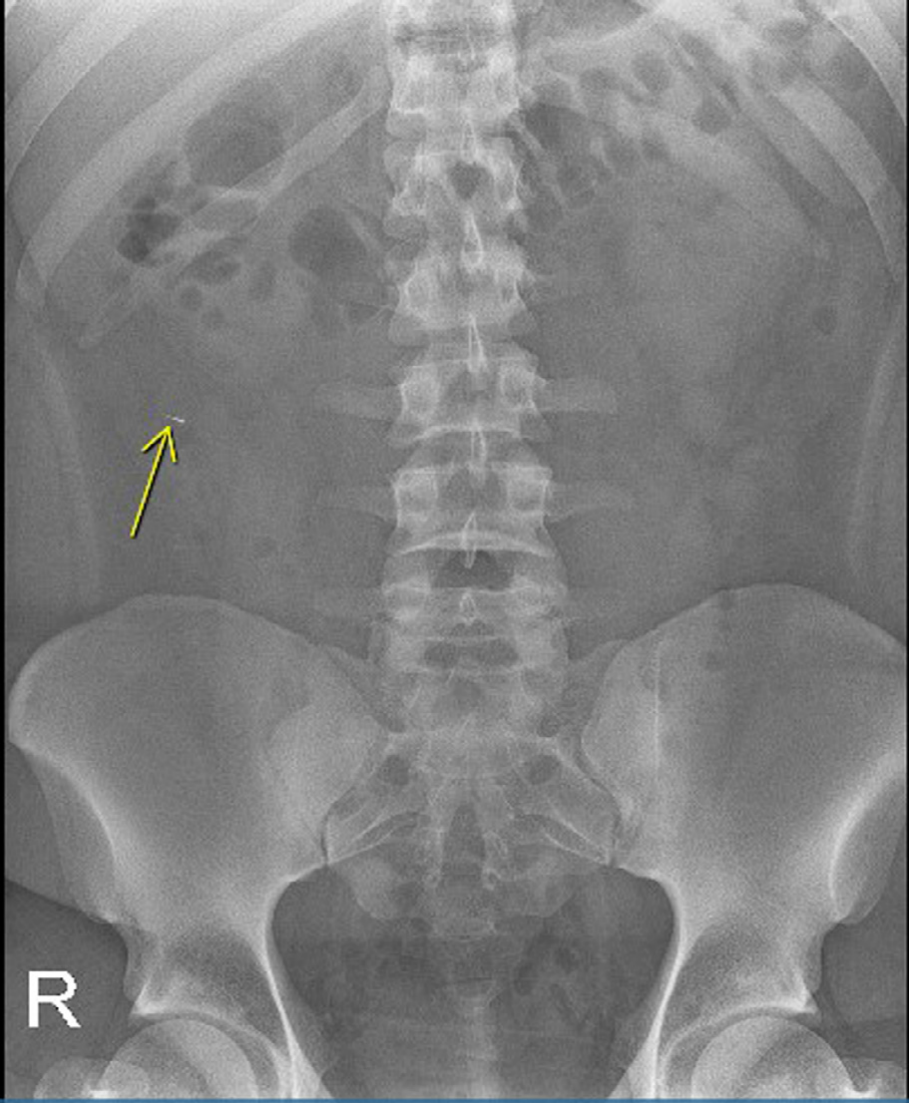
X-ray of abdomen demonstrating migrating foreign object. A repeat X-ray of the kidneys, ureters and bladder revealed redemonstration of a linear radiopaque foreign body in the right mid-abdomen, migrating likely into the ascending colon (arrow).
